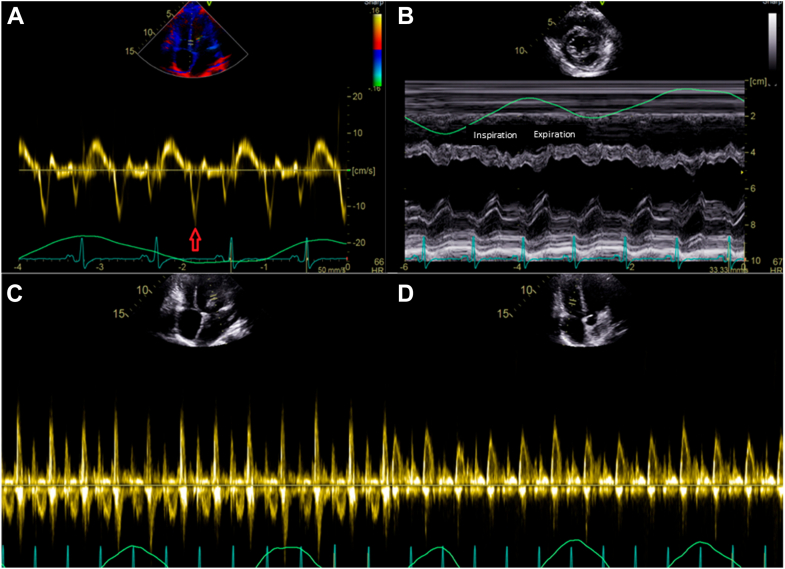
Case 1. Two-dimensional TTE, apical 4-chamber (A, C, D) and parasternal short-axis (B) views with tissue Doppler (A), M-mode with respirometer (B), and pulsed-wave Doppler of the tricuspid valve (C) and mitral valve (D), demonstrates a supranormal septal e’ (*arrow*, 16 cm/s), respirophasic movement of the interventricular septum due to exaggerated interventricular dependence (fulfilling the 2 criteria in the “2 + 1” framework), and a lack of significant respiratory variation across the mitral valve (18%) and tricuspid valve (33%).
